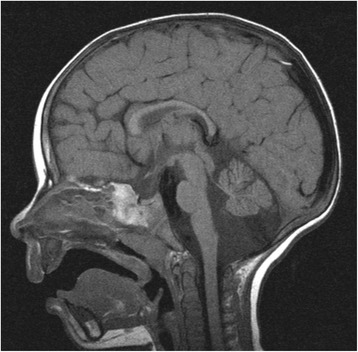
Cerebral MRI revealing an isolated hypotrophic caudal cerebellar vermis
